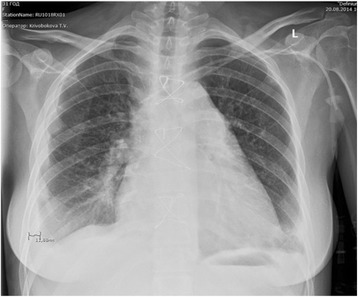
Chest X-ray: dynamics of reperfusion pulmonary oedema after pulmonary thromboendarterectomy, the 18^th^ day of ECMO; Figure demonstrates dynamics of reperfusion pulmonary oedema
